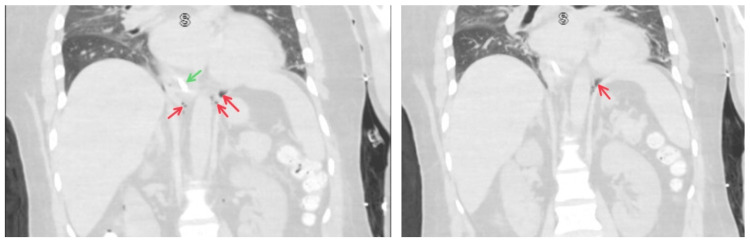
CT abdomen showing air dissecting down from thorax to abdomen Air (marked by red) can be seen along the descending aorta and esophagus (marked by green with a nasogastric tube in place).
